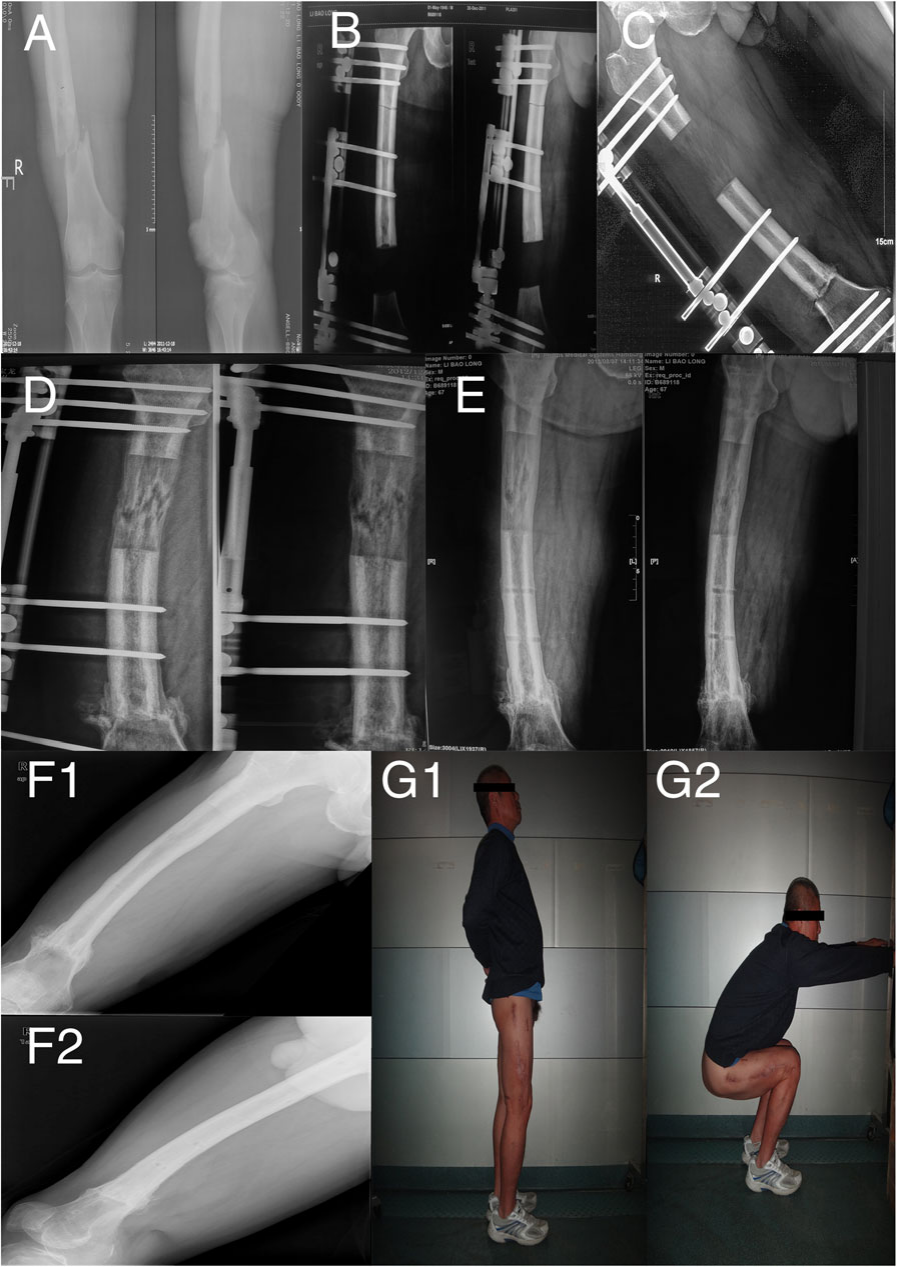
Sixty-year-old male, suffered a 2-year-long postoperative infection after fracture of left femoral shaft. a The X-ray showed refractures occurred 2 years later; b the patient was given the debridement of lesions, single-arm external fixator, and bone transport. The postoperative presentation of the X-ray demonstrated a 10-cm-long bone defect of femur. c The 5-month-later presentation of the X-ray demonstrated the femur length became normal. d The 1-year-later presentation of the X-ray demonstrated the bone grew well in the region of distraction osteogenesis and the docking site healed well. e The 18-month-later presentation of the X-ray with external fixator removed. f The 4-year-later X-ray presentation showed no infection recurred. g The patient showed a good function of flexion and extension of knee joint
